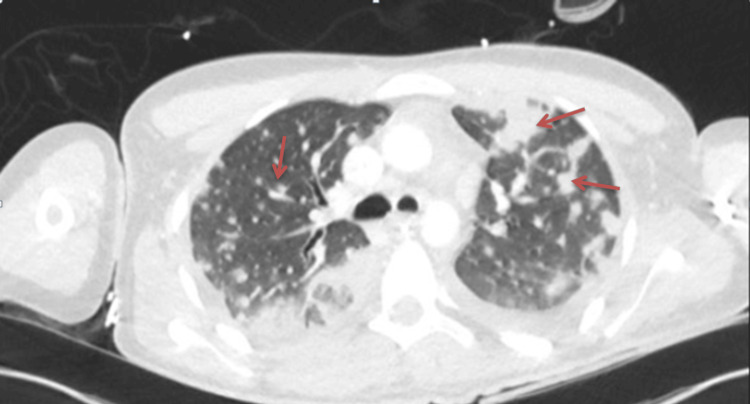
Computed tomography (CT) of the chest. CT of the chest demonstrating bilateral cavitating consolidation and septic emboli (red arrows).
